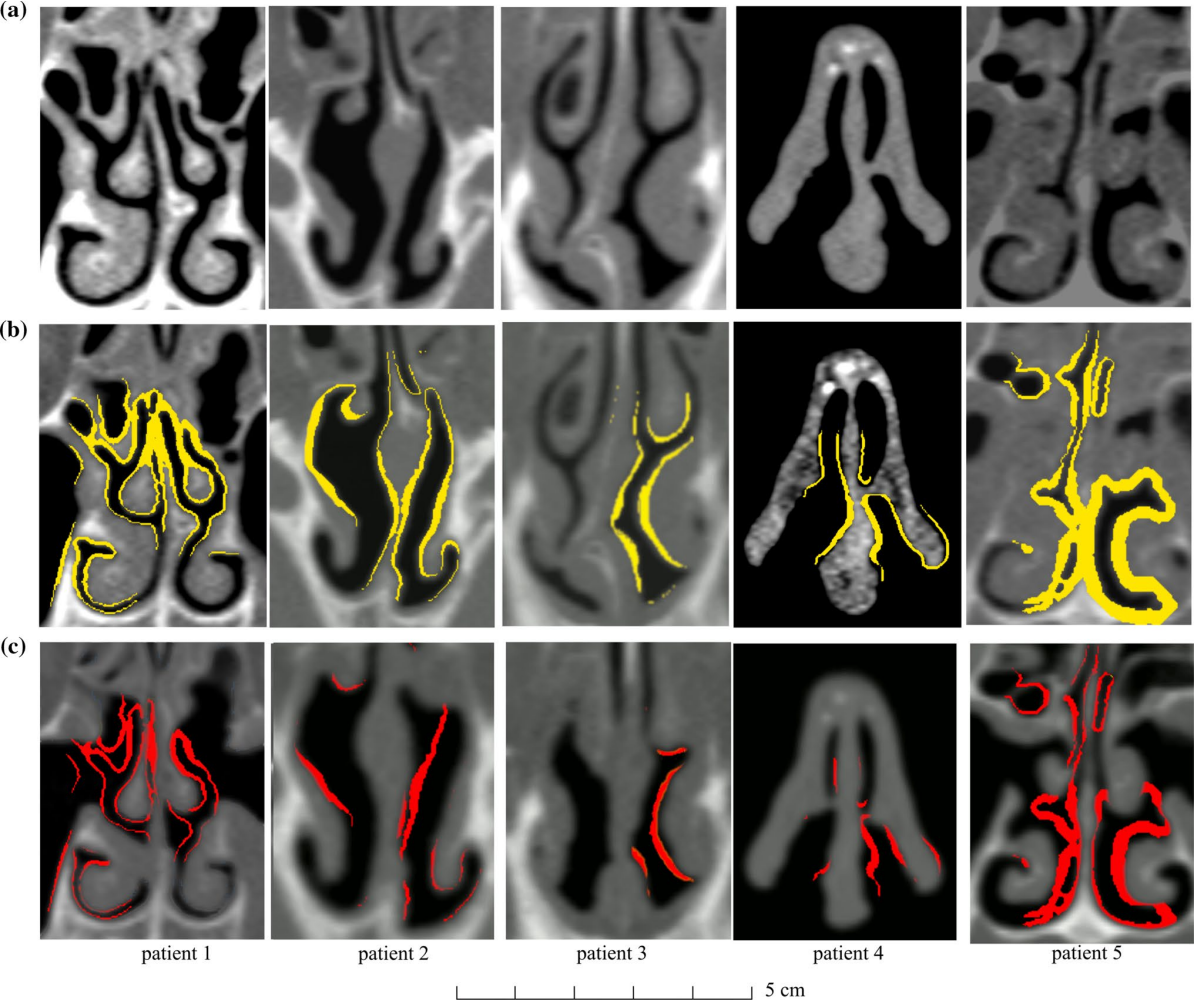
Coronal scans of nasal cavities. a Pre-surgery CT data. b Pre-surgery CT data and calculated resection areas in yellow. c Subtraction of registered post-surgery and predicted resection CT data, i.e. actually resected volumes (*red*)
